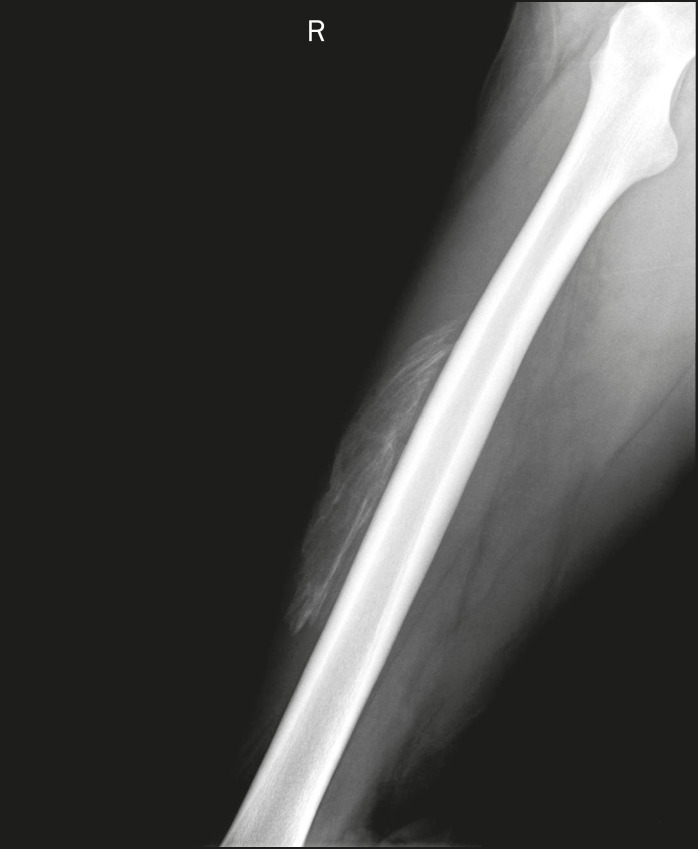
Muscle calcifications in myositis ossificans. Lateral X-ray of the thigh showing calcifications with cortical and medullary spaces outlining the fibers of the muscle belly.
